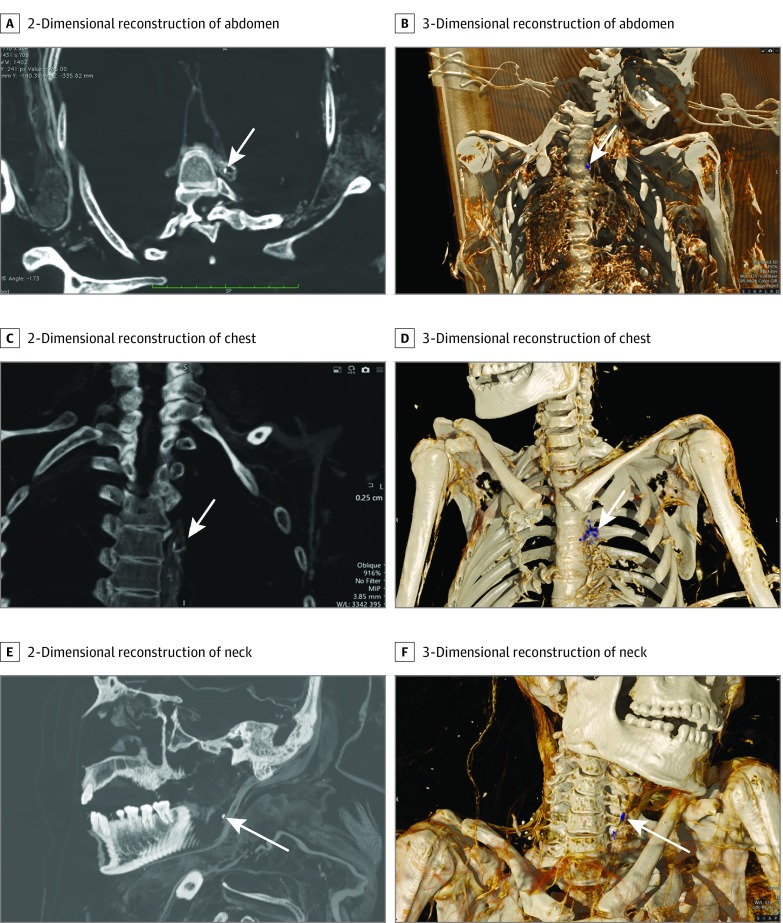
Computed Tomography Images Showing Calcified Atherosclerotic Plaques Arrows indicate calcified atherosclerotic plaques.
